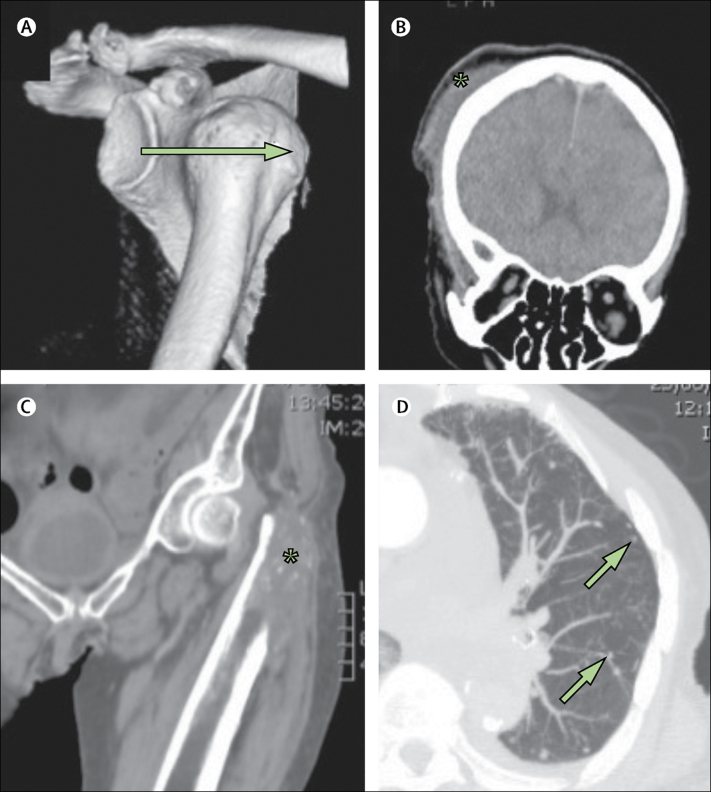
Images from post-mortem CT with targeted coronary angiography in two cases of trauma (A, B) An 86-year-old woman who was found dead in the rear doorway to her home on a cold day in February. PMCTA agreed with autopsy on the presence of ischaemic heart disease, but autopsy failed to report the trauma, which was potentially relevant in this case. (A) 3D-bone reconstruction with anterior dislocation of the shoulder (arrow shows the direction of dislocation). (B) Coronal brain multiplanar reconstruction image with subcutaneous haematoma (*). (C, D) A 91-year-old woman with an agreed primary cause of death of myocardial insufficiency due to aortic stenosis. However, PMCTA recorded in part 2 of the death certificate (associated conditions) an (C) acute pathological fracture of the left femur (asterisk) and (D) lung metastases (arrows), not reported on autopsy, which were thought likely to have acutely exacerbated her chronic cardiac condition. PMCTA=post-mortem CT with targeted coronary angiography.
